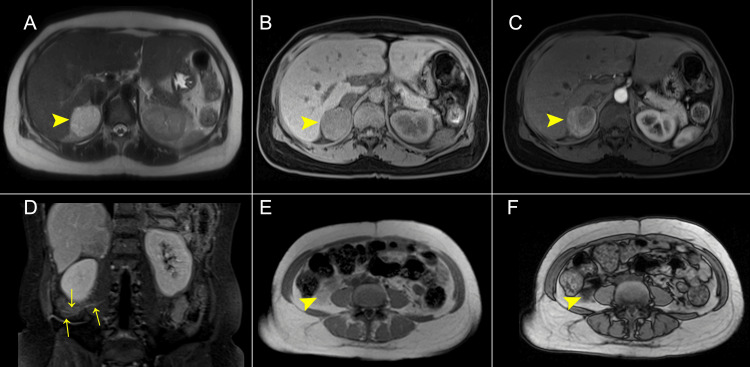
Gadolinium-enhanced abdominal MRI with non-hepatic-specific contrast. (A) T2WI sequence axial plane with markedly hyperintense signal of the right adrenal mass (light bulb sign) and (B) T1WI hypointensity signal. (C) T1FS post-contrast arterial phase axial imaging with hypervascular behavior. (D) Prominent vascular structures were present. (E) Dual-echo in-phase and (F) opposed-phase T1-weighted imaging showing loss of signal of the lesion inferior to the right renal pole. MRI: magnetic resonance imaging; T1WI: T1-weighted imaging; T1FS: T1 fat suppression; T2WI: T2-weighted imaging.
